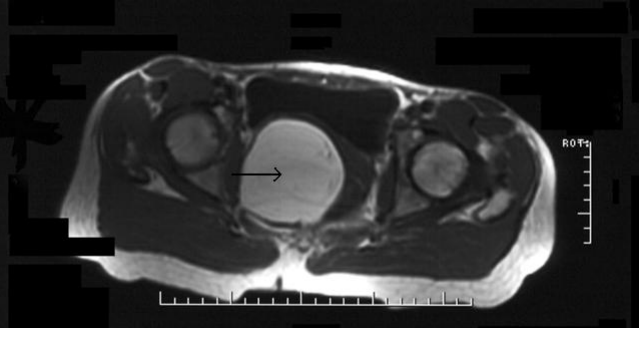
Transverse T1-weighted MRI section (arrow indicating tumour).
